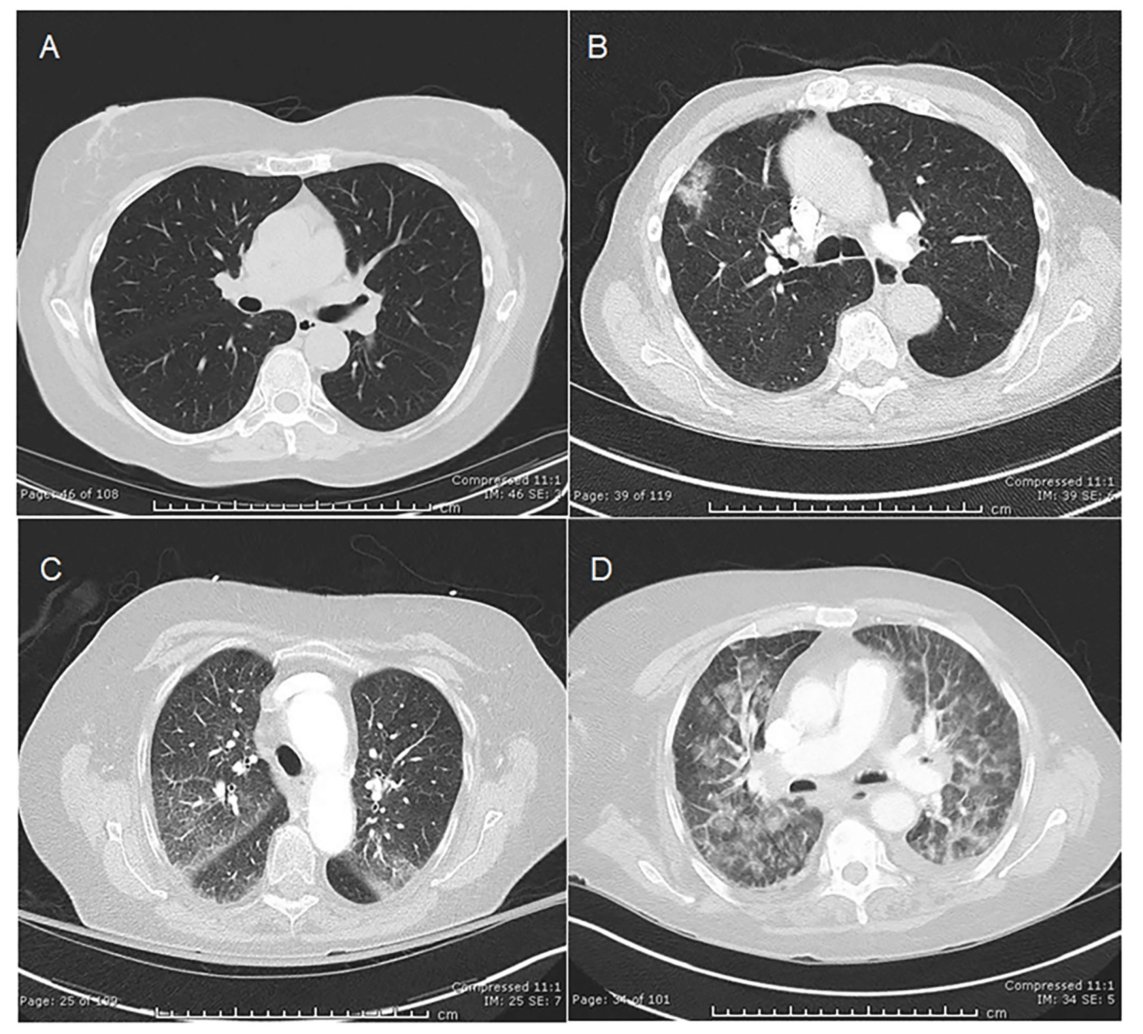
Representative chest CT scans in COVID-19 patients. A) Normal, B) Mildly abnormal, C) Moderately abnormal, and D) Severely abnormal
